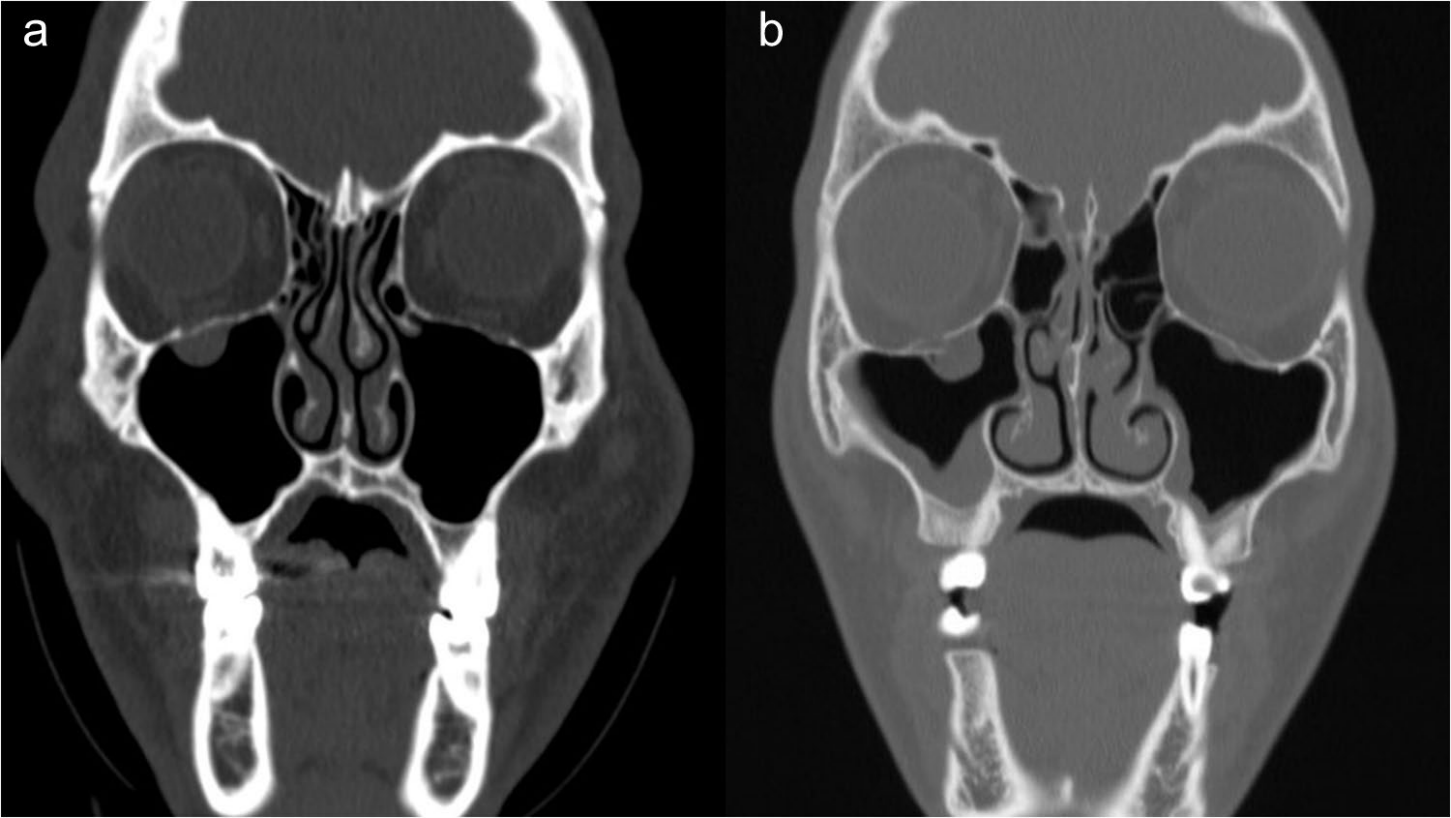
Preoperative CT scan, coronal view: a OFTF type 1a or linear fracture; b OFTF type 1b or hinged fractures according to Gerbino et al.
